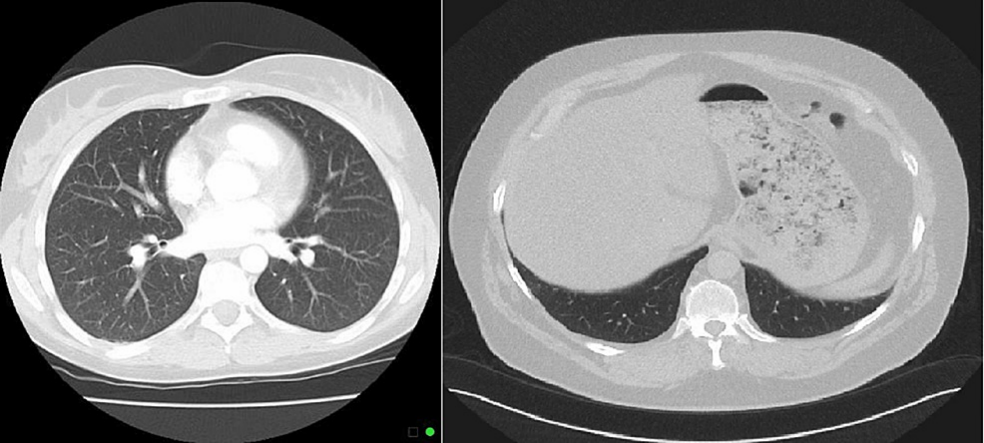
Axial CT scan of the chest with lung window of the same patient after two weeks.
